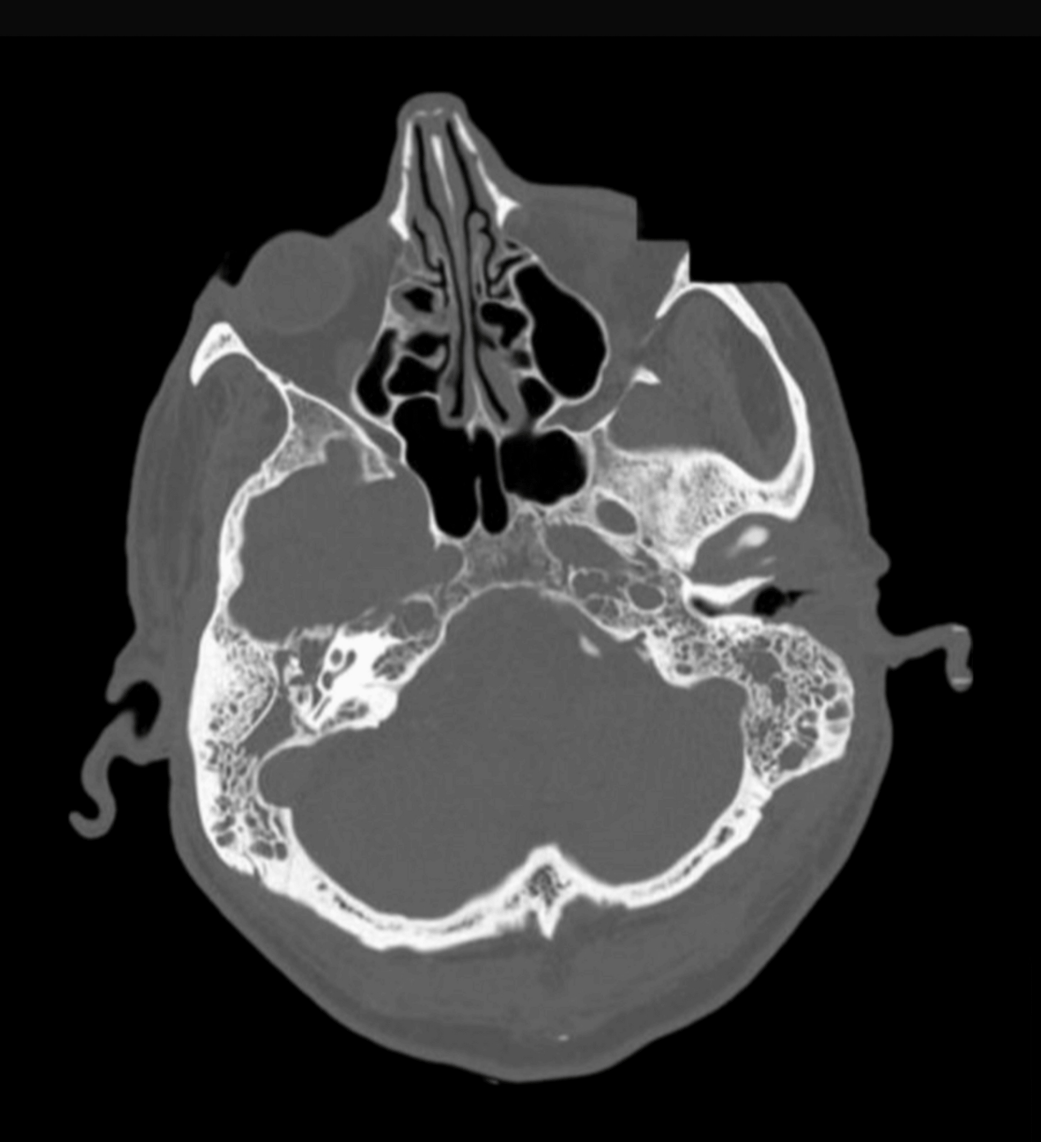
CT of the head showing tissue destruction of the right petrous apex and skull base.
